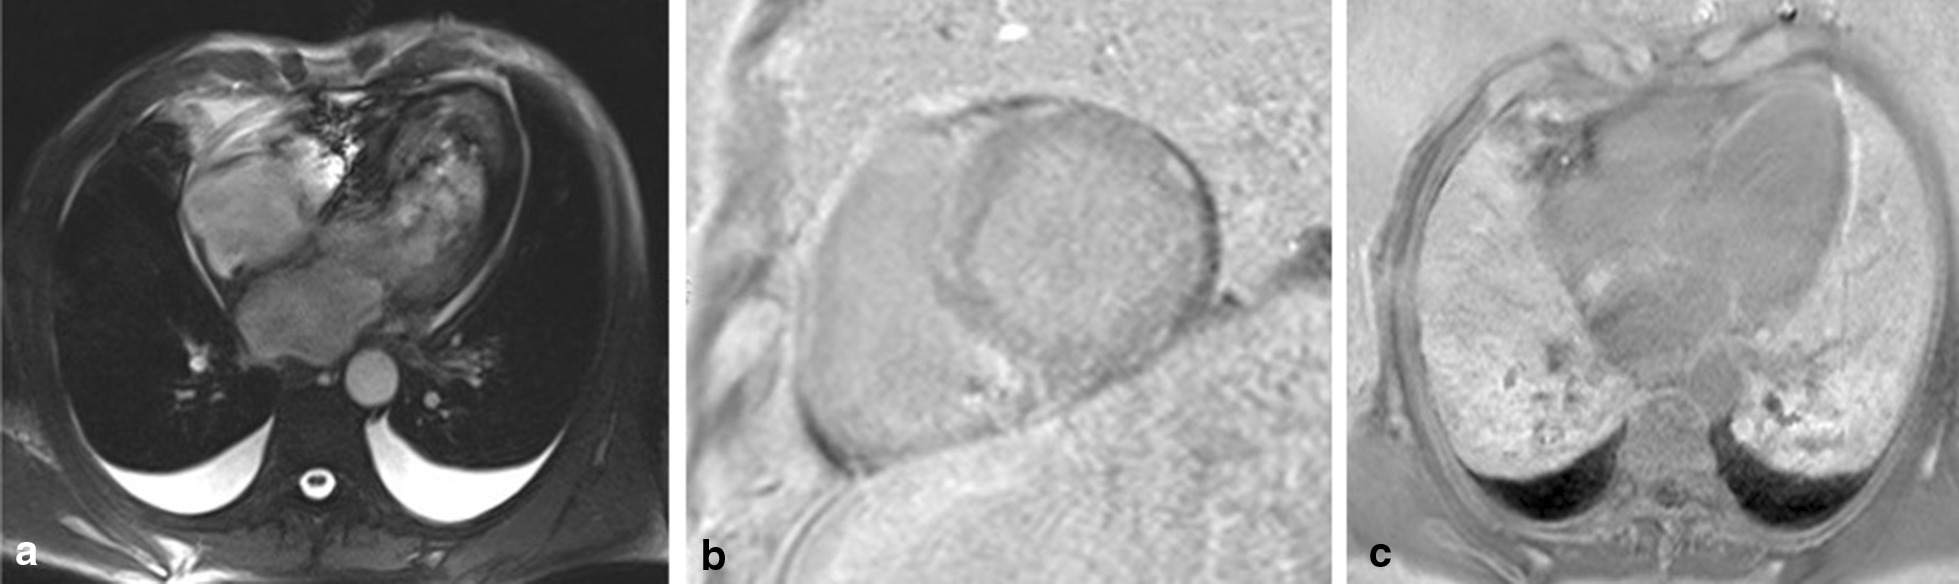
Cardiac magnetic resonance findings. Cardiac magnetic resonance images in cine long axis view (a) and post contrast long axis (b) and short axis view (c). Despite pacemaker artifacts, a hypertrophic left ventricle with diffuse myocardial late gadolinium enhancement is appreciable
